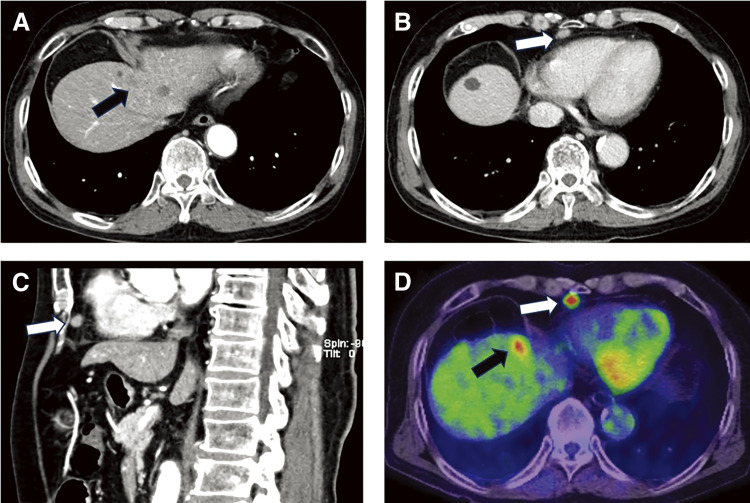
Contrast-enhanced CT and FDG-PET. (A) CT showed a 15-mm-large, low-density lesion in S4 of the liver. (B) CT demonstrated the enlarged lymph node in the anterior mediastinum (axial view). (C) Anterior mediastinal lymph node (sagittal view). (D) The accumulation of FDG in the lymph node and the hepatic lesion was observed by PET. Black arrow: hepatic lesion; white arrow: metastatic lymph node
